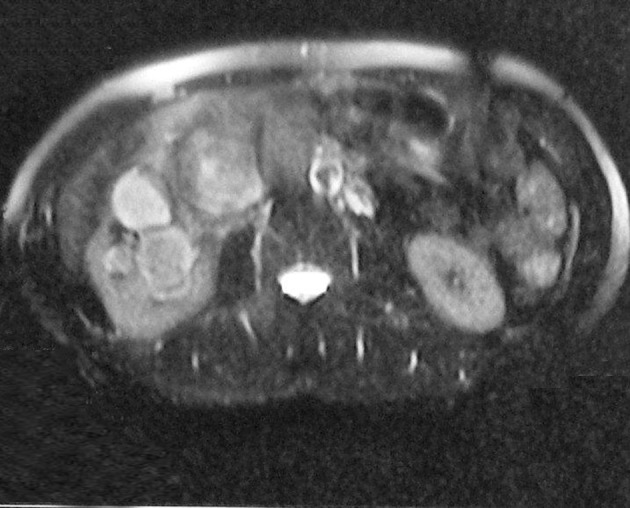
Fat suppressed axial T2-weighted MR image shows hyperintensity of the mass compared to the surrounding muscle tissue showing heterogeneous signal intensity.
